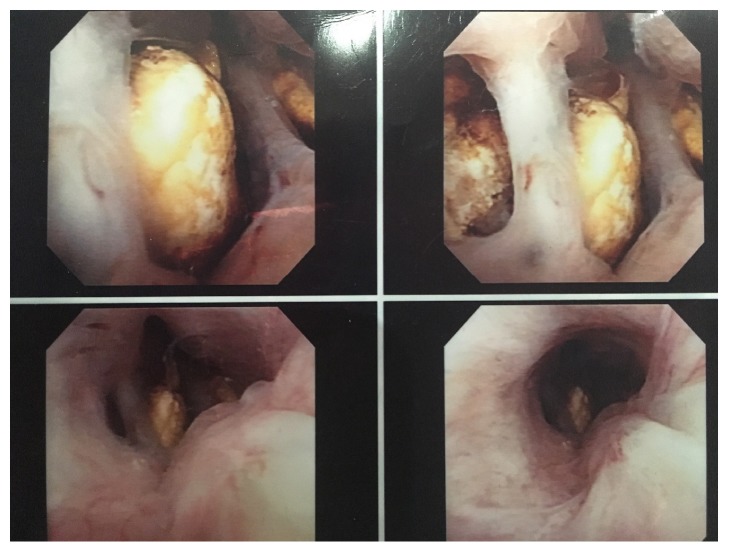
Axial view of CT abdomen and pelvis noting a large burden of prostate stones.
